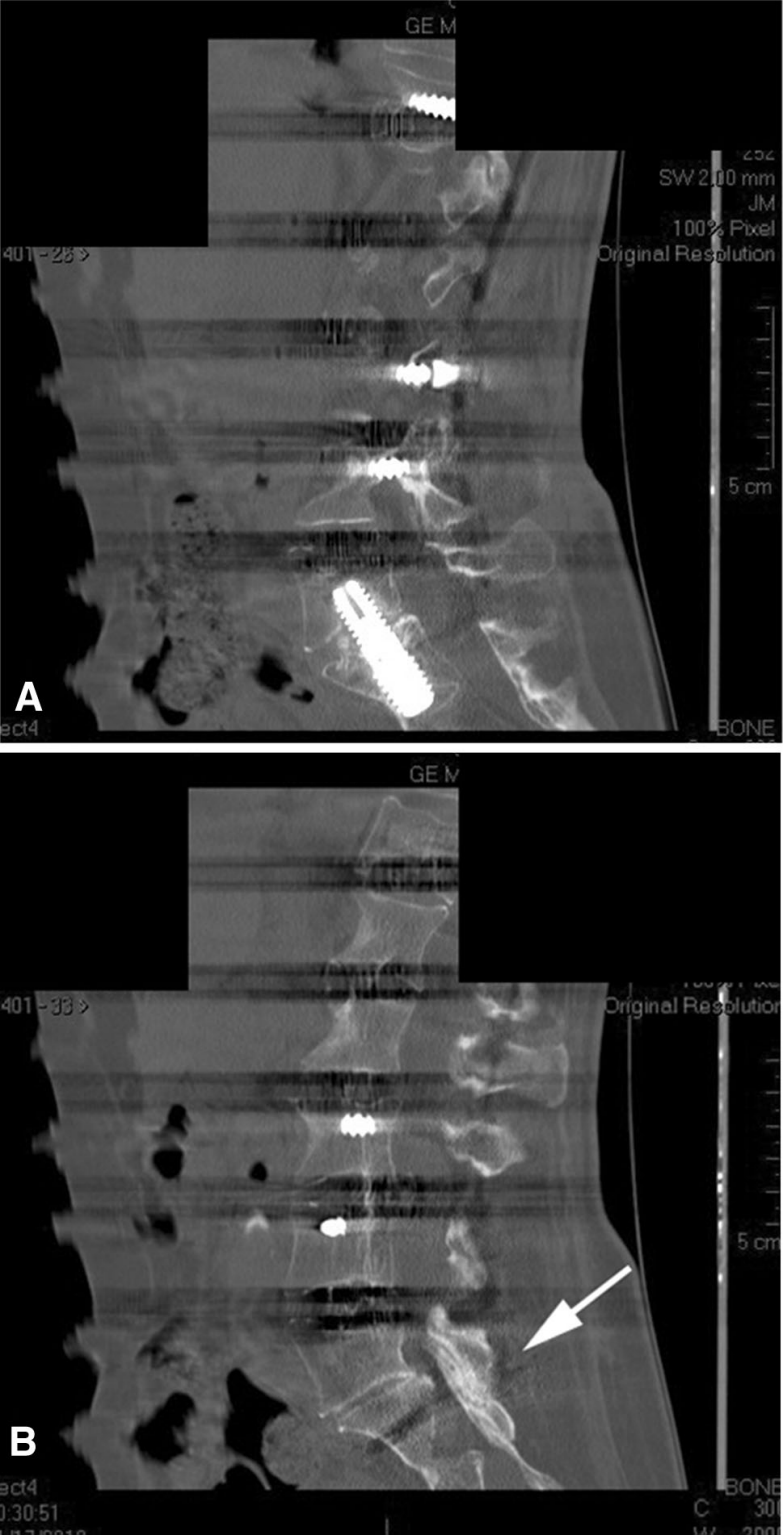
Sagittal CT reconstructions 1 year after minimally invasive deformity reconstruction are shown. (A) A midsagittal cut shows some anterior interbody bone material. (B) An image through the L5-S1 facet shows a solid facet fusion (arrow) after use of BMP, local bone, and demineralized bone matrix for fusion.
